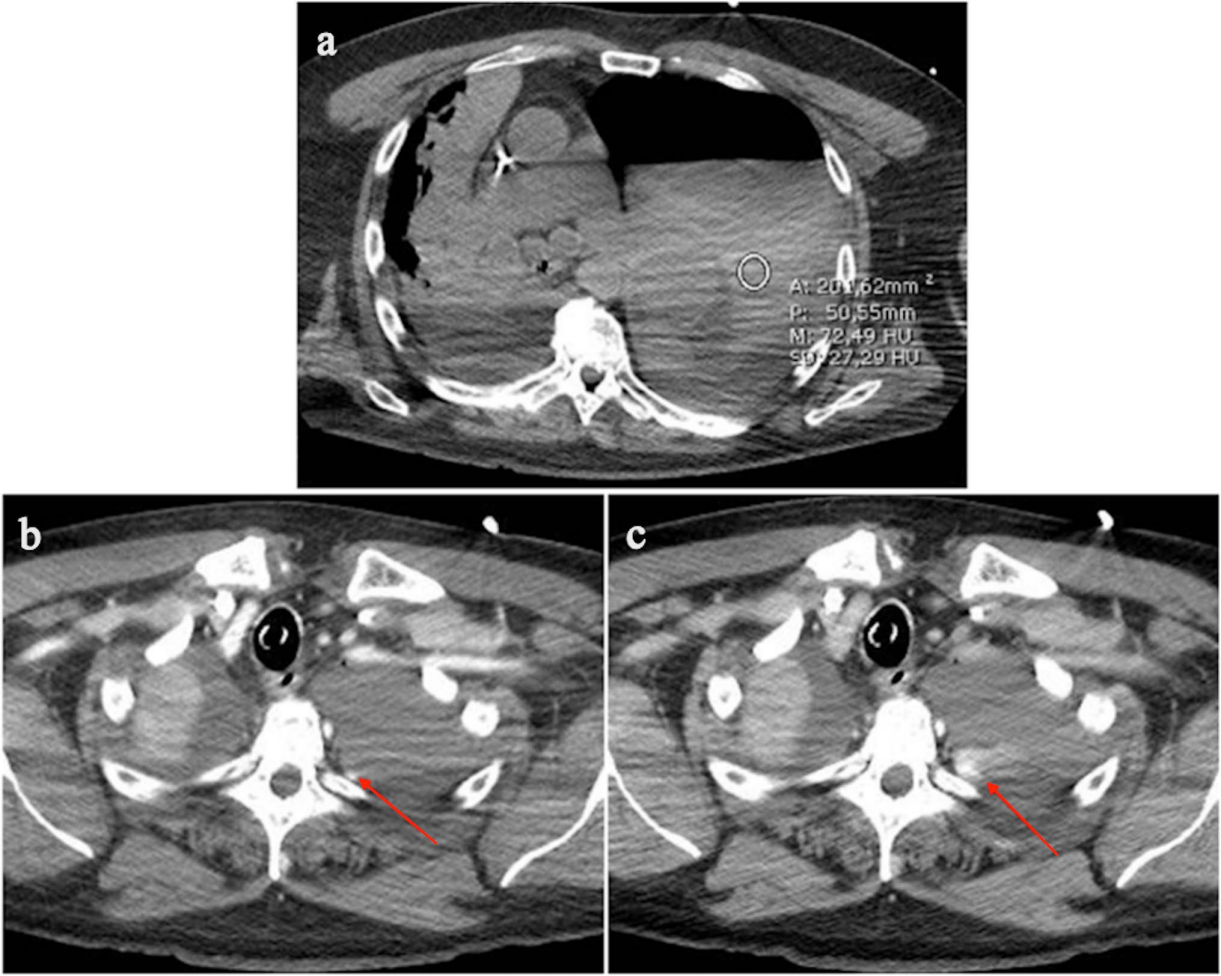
a Precontrast chest CT image showing hemopneumothorax in a COVID-19 patient with ECMO, rapidly decreasing hemoglobin level and hypotension. Post-contrast CT images in the b arterial (arrow) and c venous phase show active bleeding (arrow)
